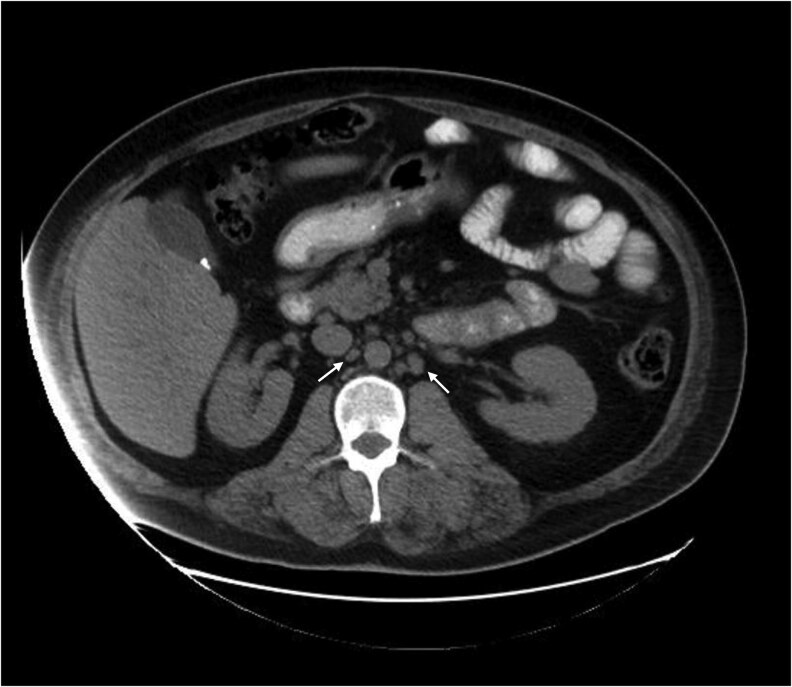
CT abdomen scan revealed mildly prominent retroperitoneal, aortocaval, and para-aortic lymph nodes (arrows).
